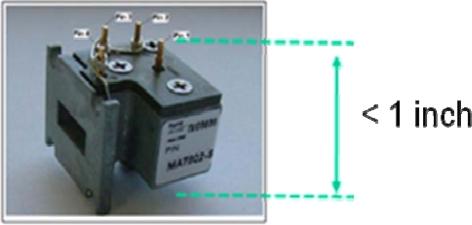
Miniature M/A-COM MACS-007802-0M1RSV K-Band Doppler transceiver [7].
